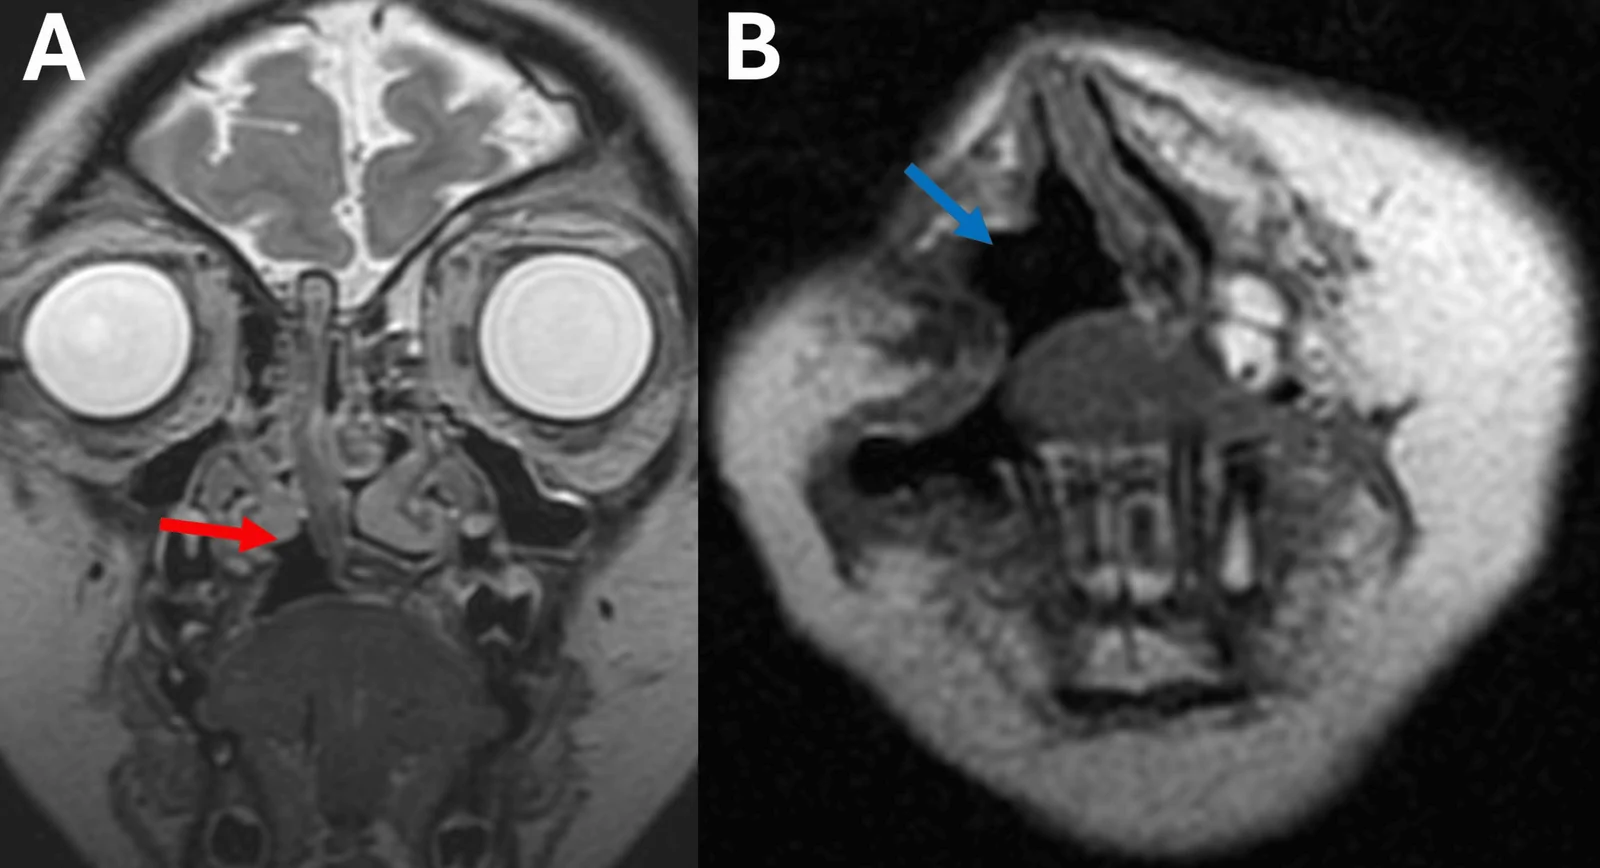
MRI images demonstrating cleft palate and cleft lip Coronal T2-weighted MRI image in the plane of the globes (A) shows cleft palate (red arrow). Coronal T2-weighted MRI image in the anterior plane (B) shows the cleft lip (blue arrow).
